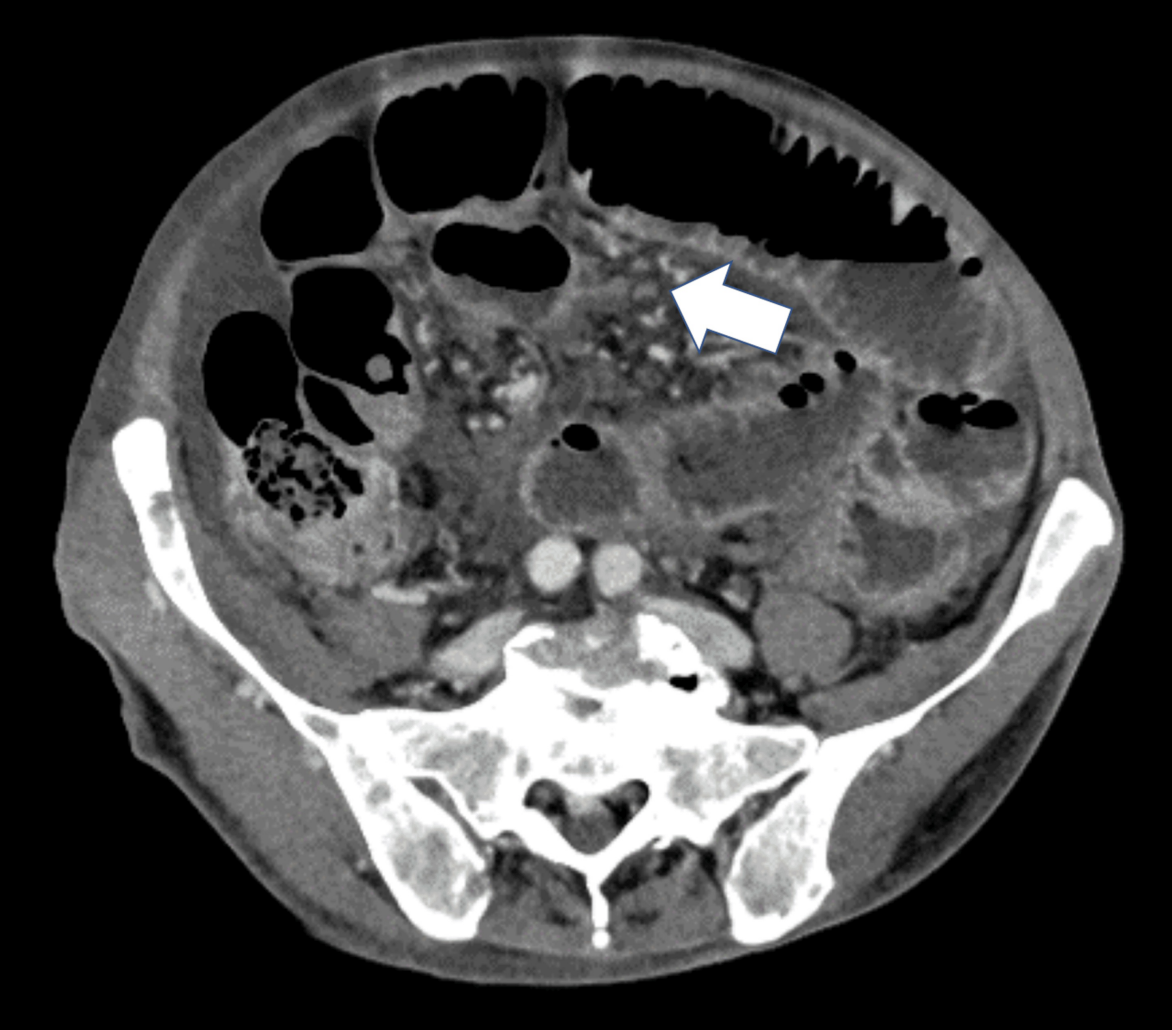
Abdominal CT Image A contrast-enhanced CT of the abdomen shows numerous peritoneal nodules (arrow) and findings indicative of ileus.
